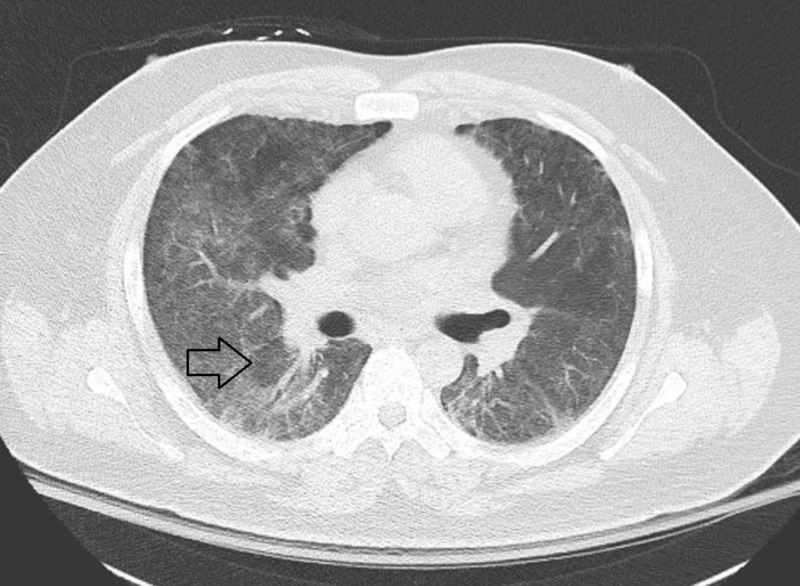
CT chest without contrast: Acute respiratory distress syndrome (ARDS) in ANCA-associated vasculitis (AAV), 10 days post VV-ECMO with radiographic improvement of lung disease and evidence of residual basal interstitial lung disease (ILD). ANCA: Anti-nuclear cytoplasmic auto-antibody; VV-ECMO: Veno-venous extracorporeal membrane oxygenation.
